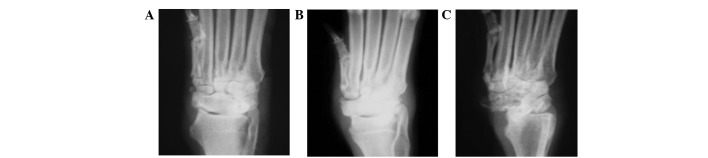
X-ray images. No marked changes in sclerotin were found in weeks (A) 3 or (B) 6 after surgery. (C) In week 12 after surgery, the density of the lunate appeared uneven, the capsular space flattened and the lunate presented an irregular shape with an unsmooth articular surface.
